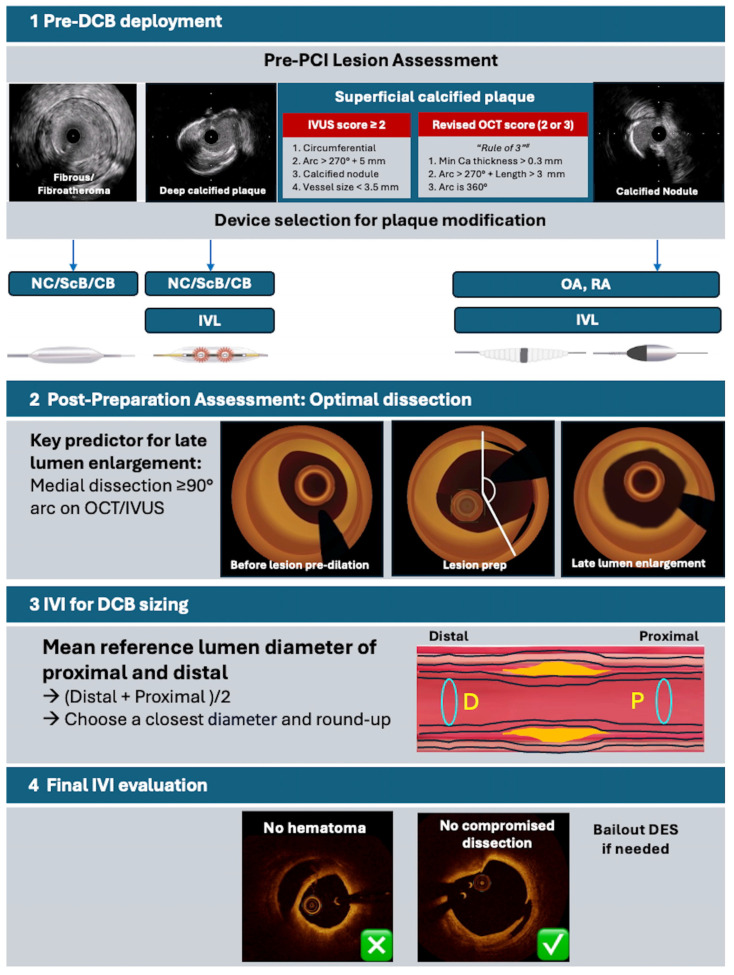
Intravascular imaging-guided DCB treatment. Abbreviation: CB, cutting balloon; DCB, drug-coated ballon; DES, drug-eluting stent; IVI, intravascular imaging; IVL, intravascular lithotripsy; IVUS, intravascular ultrasound; NC, non-compliant balloon; OA, orbital atherectomy; OCT, optical coherence tomography; POT, proximal optimization therapy; RA, rotational atherectomy; ScB, scoring balloon; SB, side branch.
